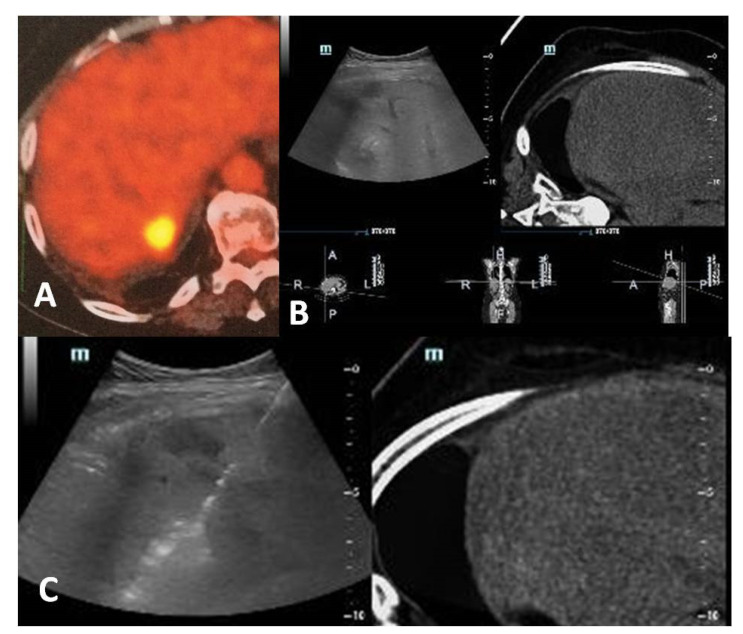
A 60-year-old, female patient with a medical record of colorectal cancer and synchronous liver metastasis for which underwent surgery and chemotherapy presented with a PET avid new hepatic lesion (A). Contrast-enhanced computed tomography scan was not possible due to prior severe allergic responses; fusion imaging was used for ablation guidance (B). A radiofrequency electrode was inserted under fusion imaging, and ablation was performed with no need for iodinated contrast medium (C).
